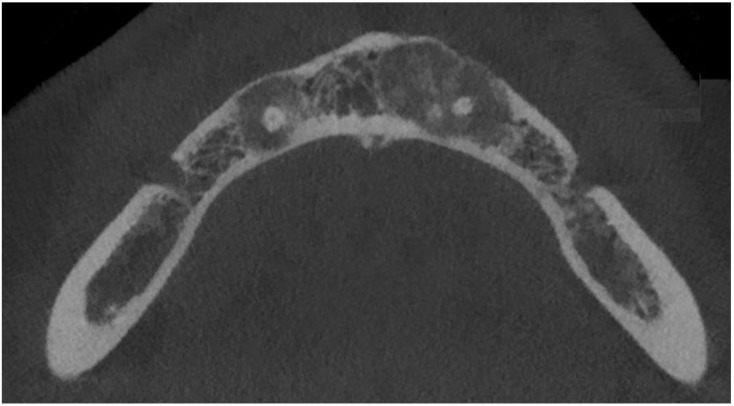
CBCT-axial view on COD in the anterior mandibular base. Most common occurrence side of COD.
